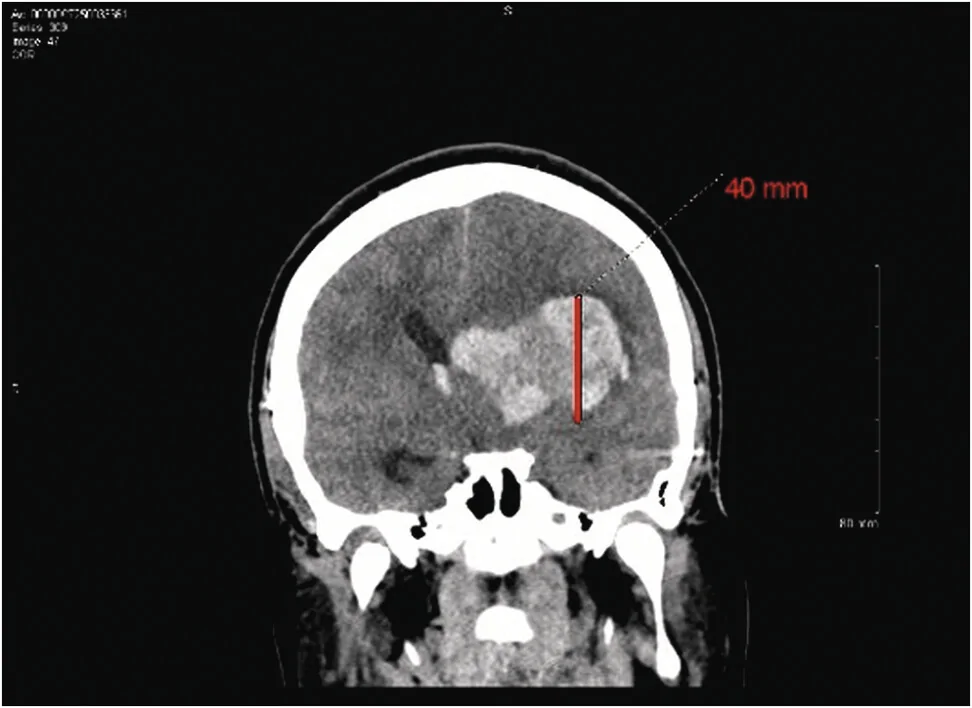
Computed tomography (CT) angiography of the brain in Case 2 demonstrating active arterial extravasation within the left cerebral hemisphere, consistent with ongoing hemorrhage.
